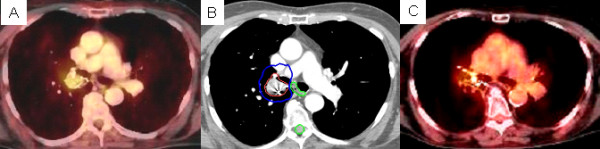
Right hilar metastasis treatment planning PET/CT with a tumor SUV_max _of 9.6 (A), planned radiation dose distribution (B: the planning treatment volume is shown in red and the 35 Gy isodose line in blue), and PET/CT at 6 months post-treatment (C) shows an excellent response with a tumor SUV_max _of 2.7.
